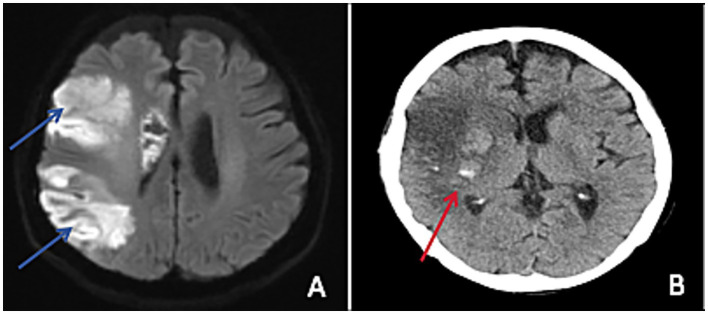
Representative case of HBC-PH hemorrhagic transformation following intravenous alteplase thrombolysis. (A) Diffusion-weighted magnetic resonance imaging (DWI) demonstrates restricted diffusion consistent with acute cerebral infarction (blue arrows). (B) Non-contrast head computed tomography (CT) shows a hyperdense intraparenchymal hemorrhage corresponding to hemorrhagic transformation (red arrows).
